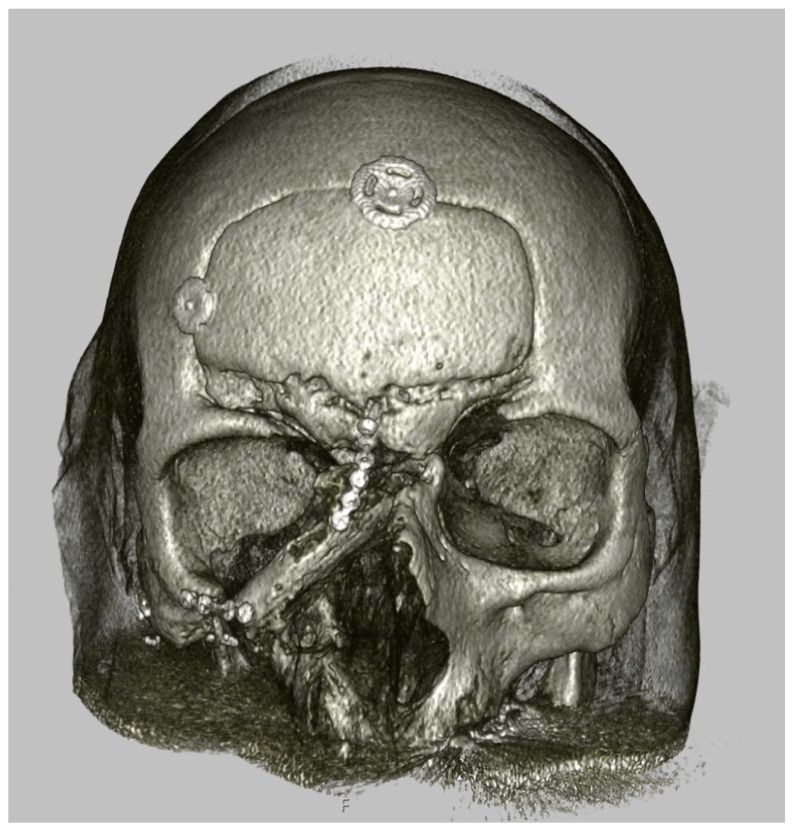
A 3D overview of the CT dataset reveals a Class V defect resulting from osteosarcoma, which was successfully reconstructed using a scapula flap with intraoral anastomoses. This reconstruction was assessed at the 36-month postoperative mark. Notably, in this specific case, the skin island was lost seven days after the surgical procedure.
